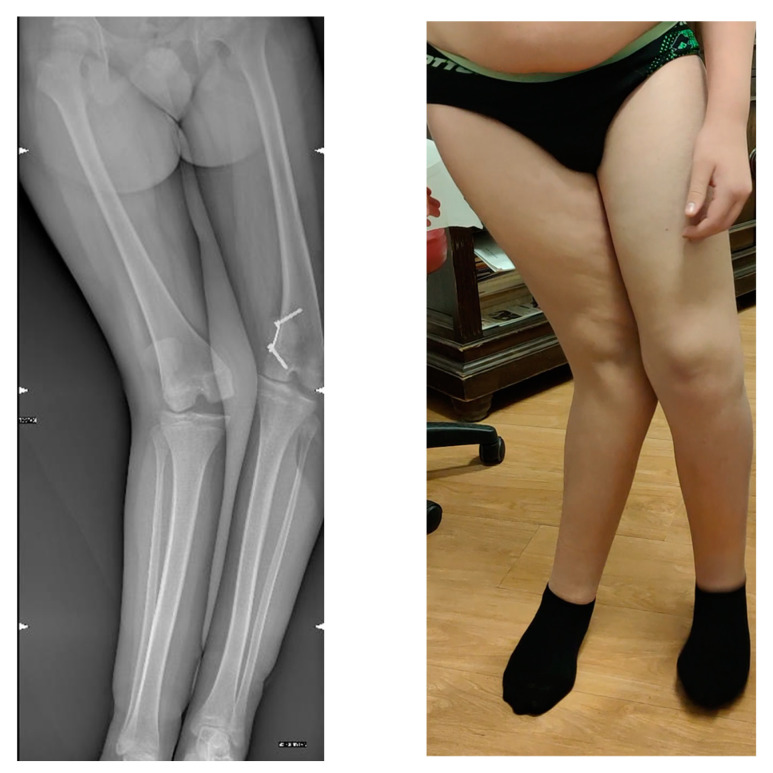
Radiographic and clinical aspects of the limbs.
